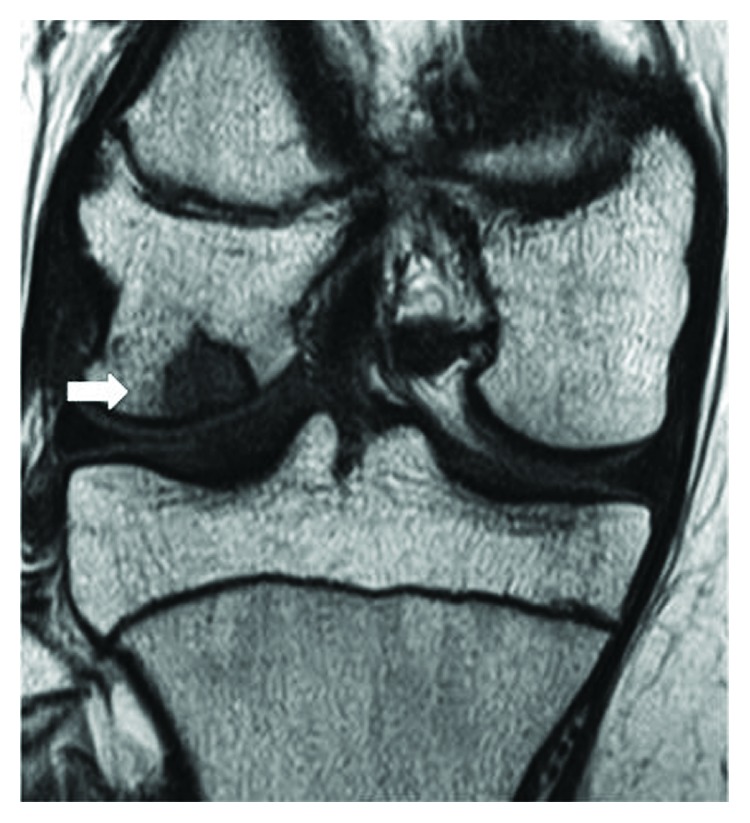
Coronal T1-weighted MR image shows a low-signal-intensity lesion (arrow).
